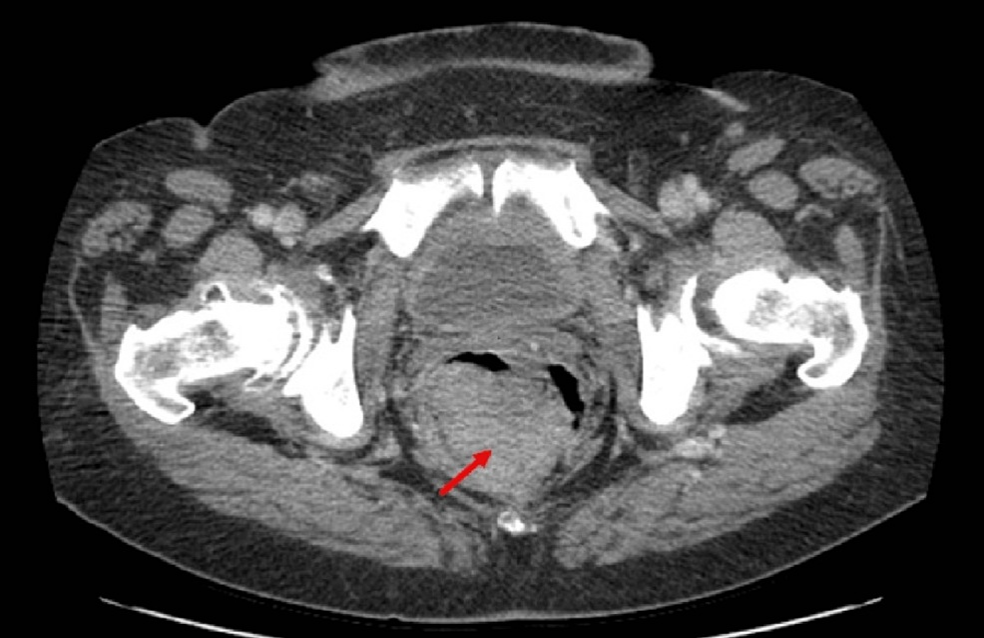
Axial contrast CT of the pelvis showing an irregular and heterogeneous rectal tumor (arrow) CT: computed tomography
